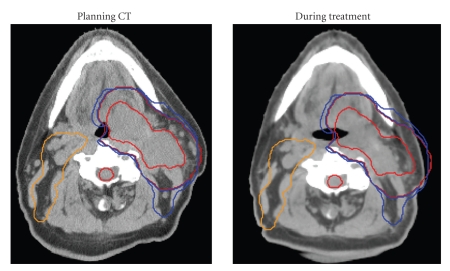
Anatomic changes can be pronounced during treatment. In this example, planning CT scan and CTV contours are shown on the left. On the right, a mid-course CT (three weeks into treatment) demonstrates significant reduction in gross tumor (thick red line). Baseline CTVs have been overlaid via rigid image registration. These match current anatomy poorly and in fact extend past the skin contour into air.
